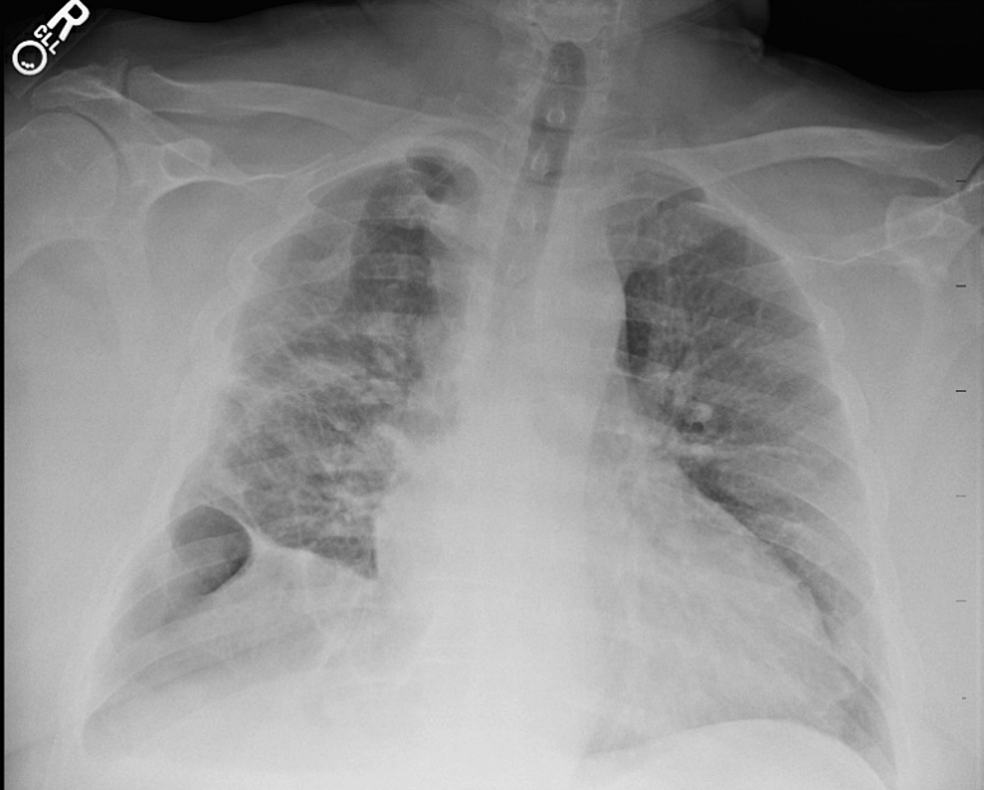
Postoperative chest X-ray at the outpatient clinic Chronic-appearing postoperative pleural parenchymal scarring laterally in the right hemithorax from the recent surgery. There is a trace residual volume of pleural fluid in the right lung base.
